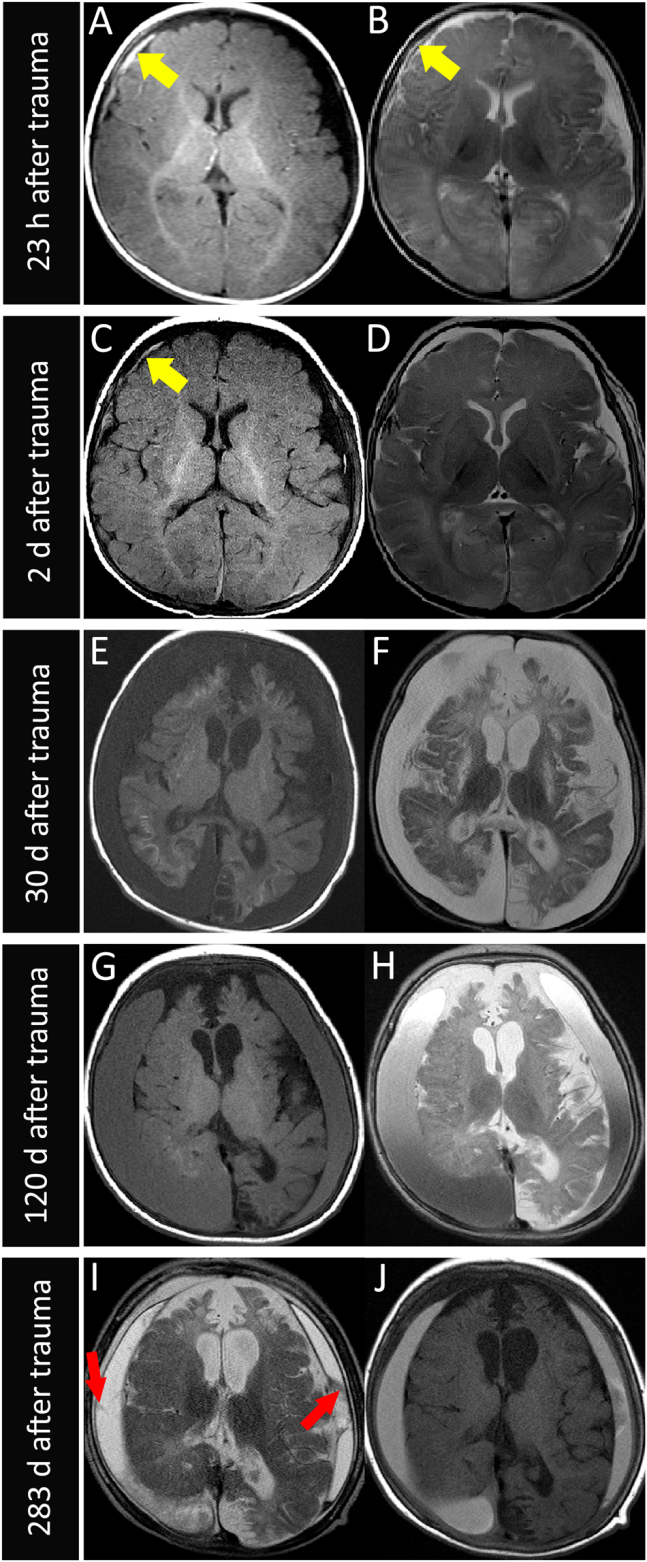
MRI scans of an initial 2-month-old girl with four follow-ups showing a typical transformation of an SDC over time. T1w imaging is shown on the left (A, C, E, G, and I), and T2w imaging is shown on the right (B, D, F, H, and J). Twenty-three hours after trauma (A + B), an SDH (yellow arrows) is detectable on the right convexity. Two days after trauma (C + D), the SDH has noticeably decreased. Thirty days after trauma (E + F), an SDHy with a CSF-like appearance, as well as defects and loss of brain parenchyma have occurred. One hundred twenty days after trauma (G + H), the SDHy has turned into an SDHHy homo with hyperintense (G) and hypointense signal (H) compared with CSF. As the patient did not undergo neurosurgery, the “new” blood component might possibly come from minimal and innocent rebleeding out of fragile new vessels of early neomembranes. Two hundred eighty-three days after trauma (I + J), a cSDH with clearly visible septa and neomembranes (red arrows) has developed
